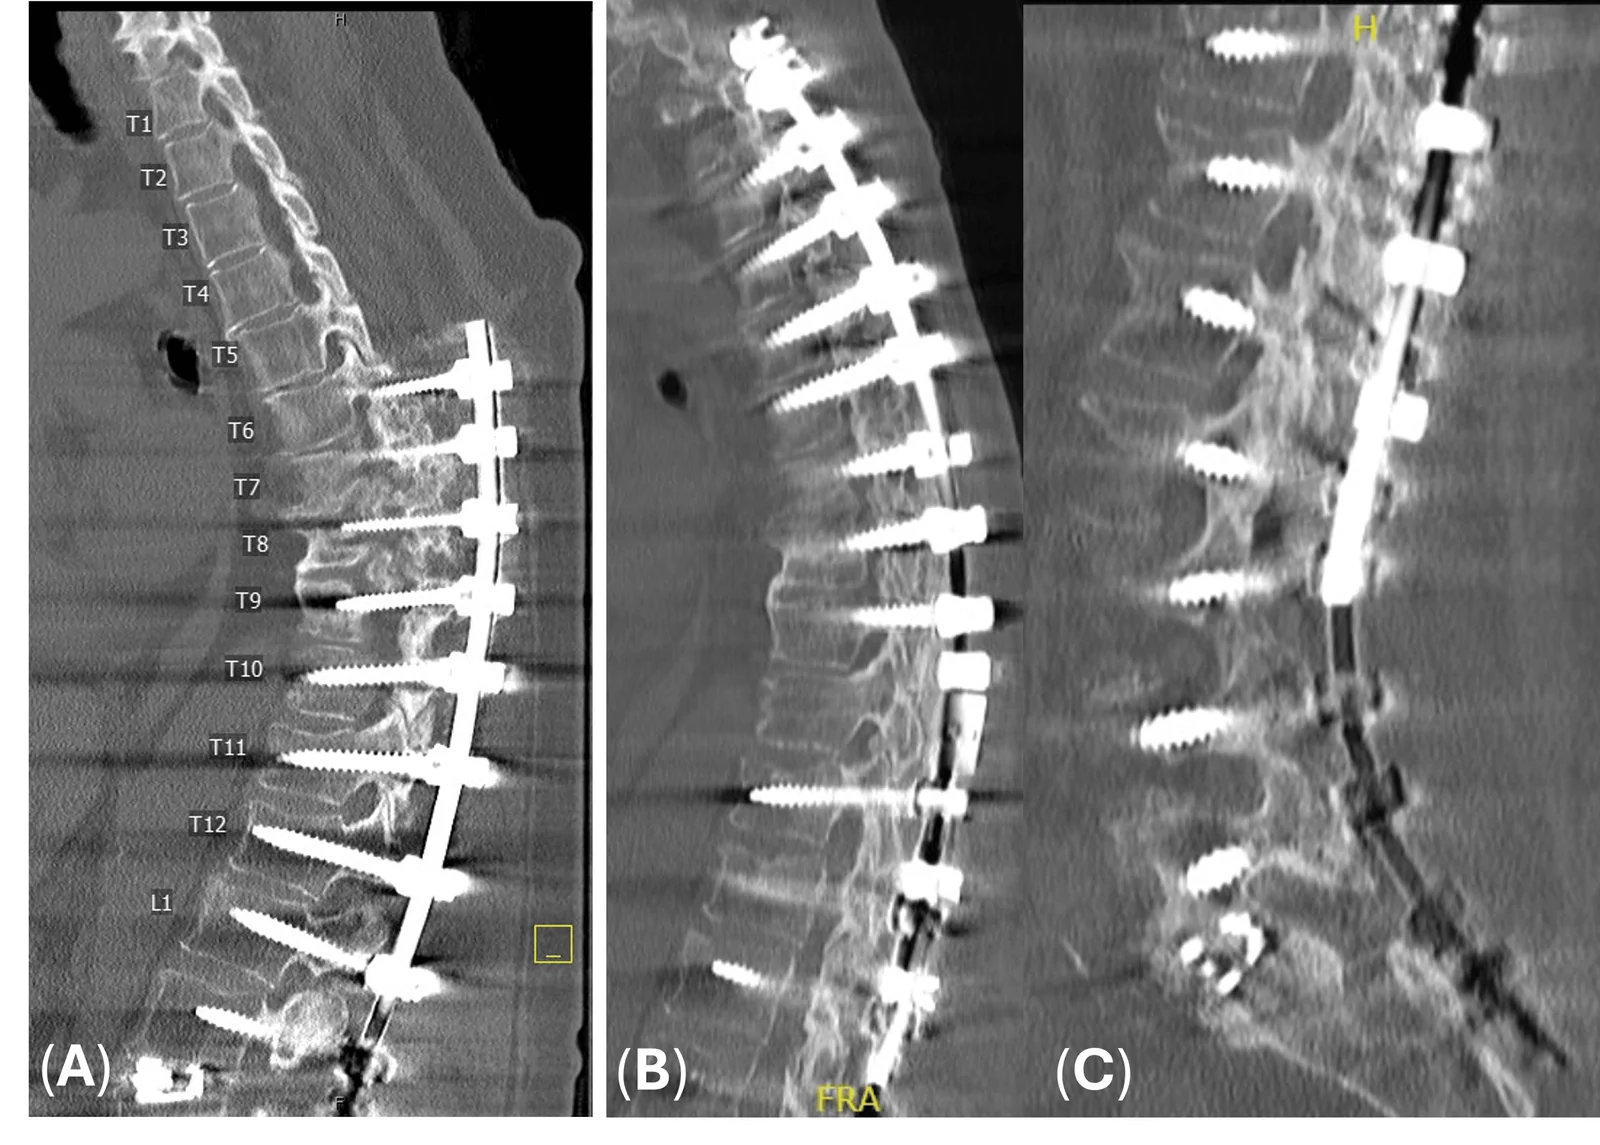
Example CT imaging from an 11-level revision posterior fusion A 61-year-old female smoker with obesity and hypertension presented with proximal junctional failure and thoracic pseudoarthrosis. Her extensive surgical history included an L3-L5 PLIF six years prior and a T6-pelvis posterior fusion with an L1-L3 TLIF eight months prior. To address the failure of the previous construct, she underwent hardware removal and a revision T2-L1 posterolateral fusion. (A) Baseline and (B, C) 12-month follow-up CTs depicting successful fusion. Image courtesy: Dr. Peter G. Passias
